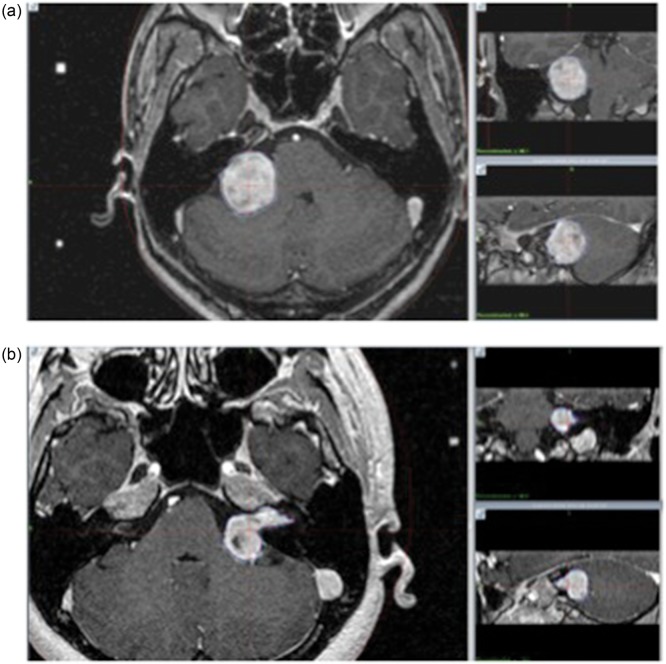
Target contours of Case 1 (a) and Case 10 (b) of vestibular schwannoma in three planes (axial, coronal and sagittal). In both cases, the delineation on stereotactic MRI (purple) and that on diagnostic MRI co-registered with stereotactic CT (blue) show good agreement.
